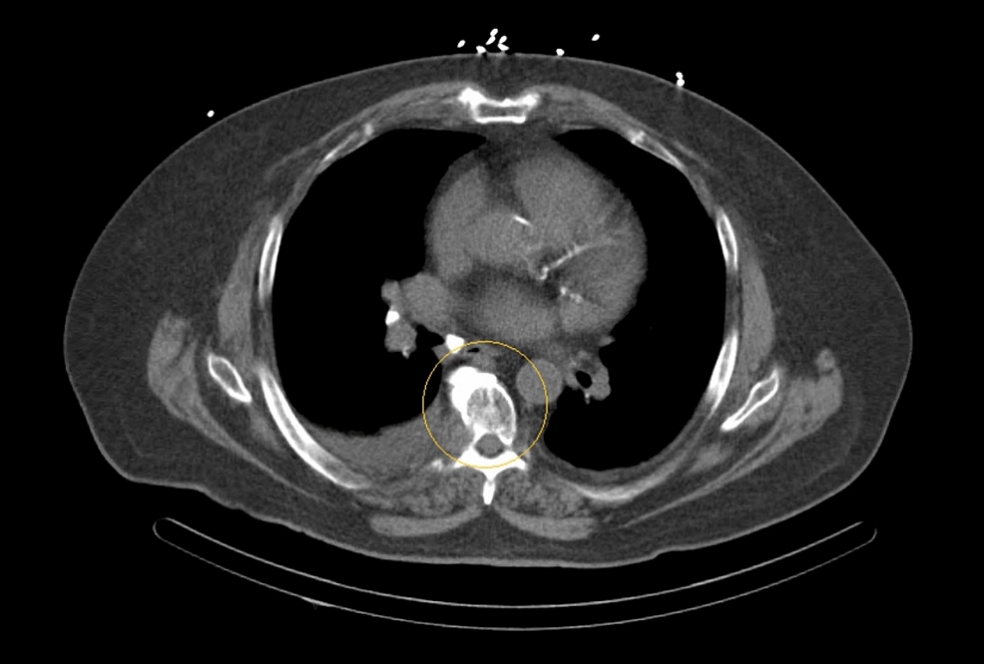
Thoracic mass revealed on the limited computerized tomography (CT) imaging of the chest.
